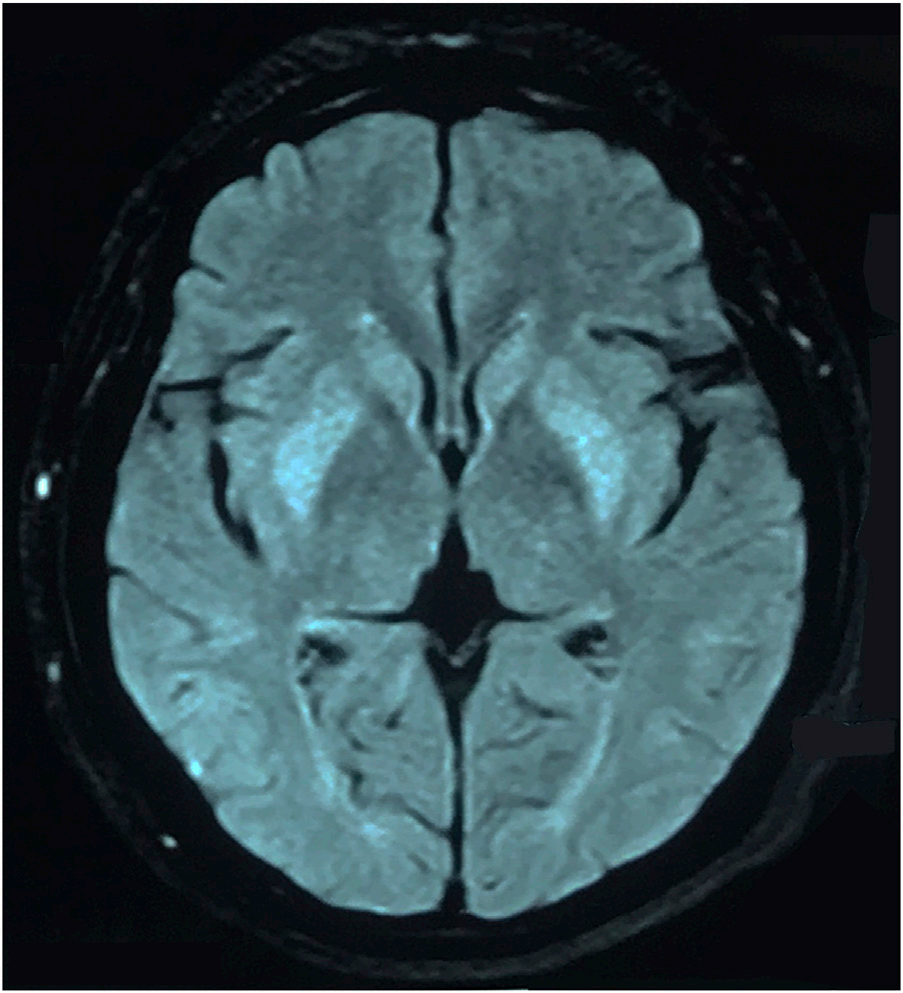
Head MRI revealed symmetric FLAIR hyperintensities of the bilateral basal ganglia.
